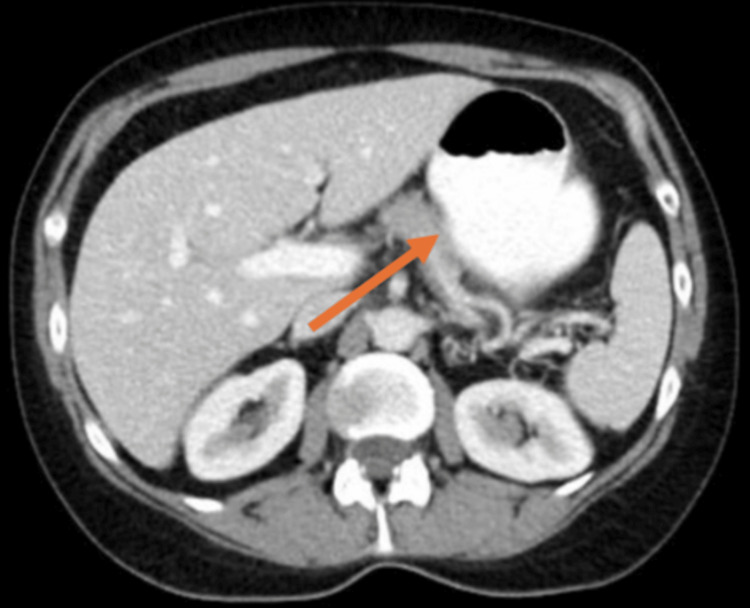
Contrast-enhanced CT of the abdomen and pelvis demonstrating an area of asymmetric mural thickening in the gastric antrum measuring 0.9 × 2.5 cm with possible reactive mesenteric lymph nodes CT: computed tomography
